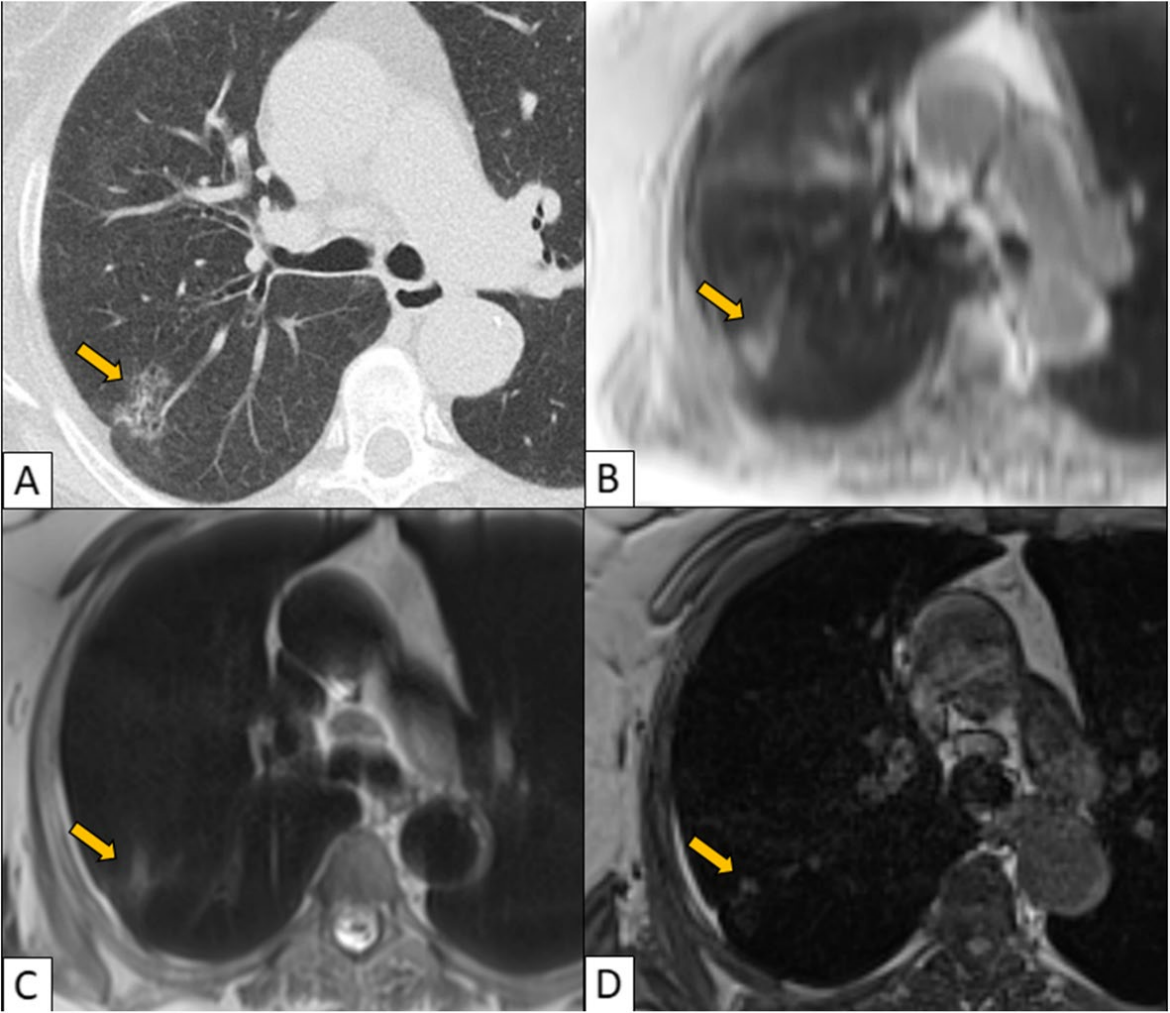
CT (A), UTE (B), HASTE (C), VIBE (D). Right upper lobe ground glass nodule is clearly detected in UTE and less well seen in HASTE. It is inconspicuous in VIBE
